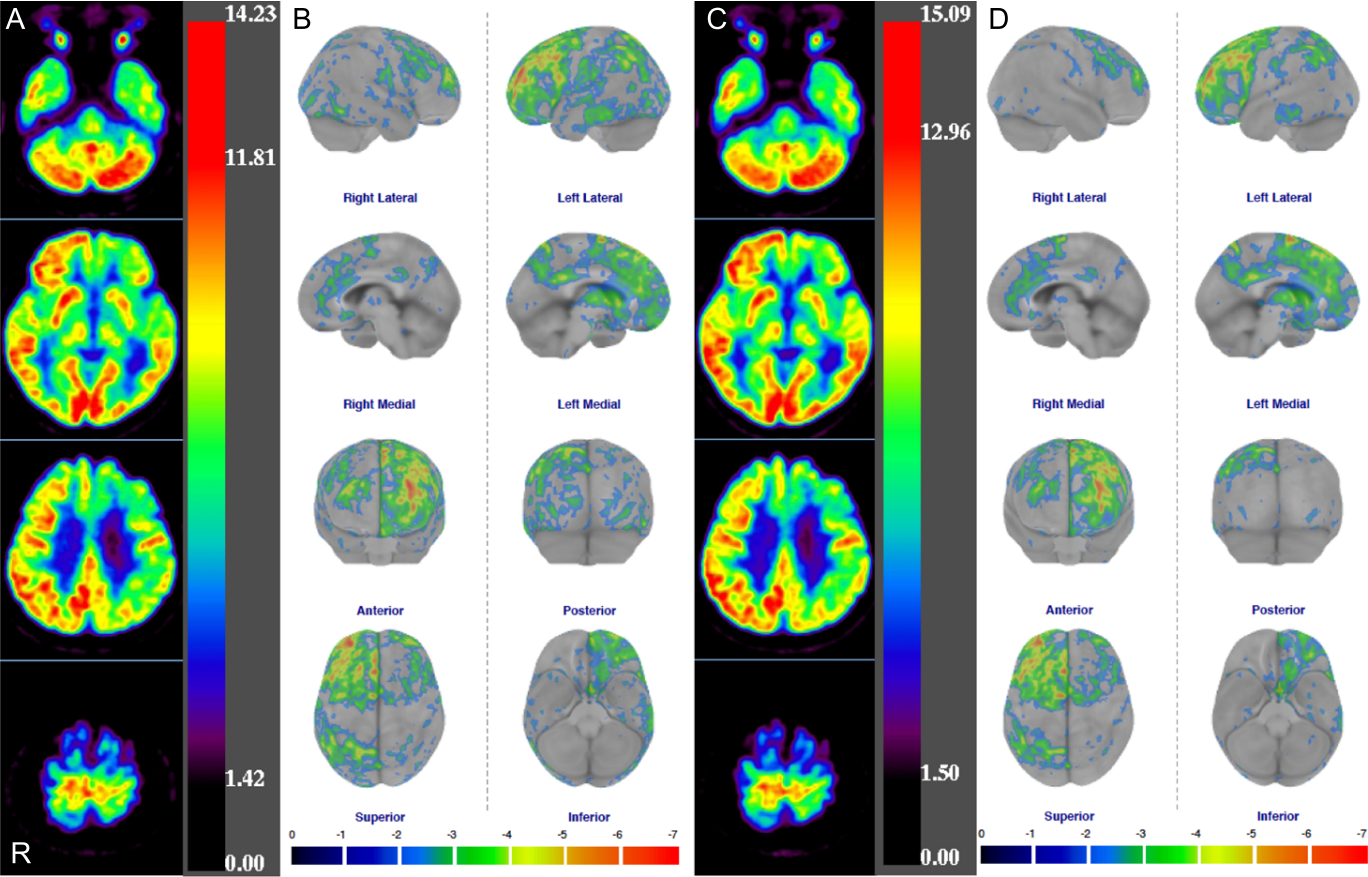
[^18^F]FDG images and *Z*-score maps derived from a 60-year-old female with suspected frontotemporal lobar degeneration. [^18^F]FDG images (a) and Z-score maps (b) in PMT-PET and [^18^F]FDG images (c) and Z-score maps (d) in SiPM-PET. PET positron emission tomography, PMT photomultiplier tube, SiPM silicon photomultiplier
